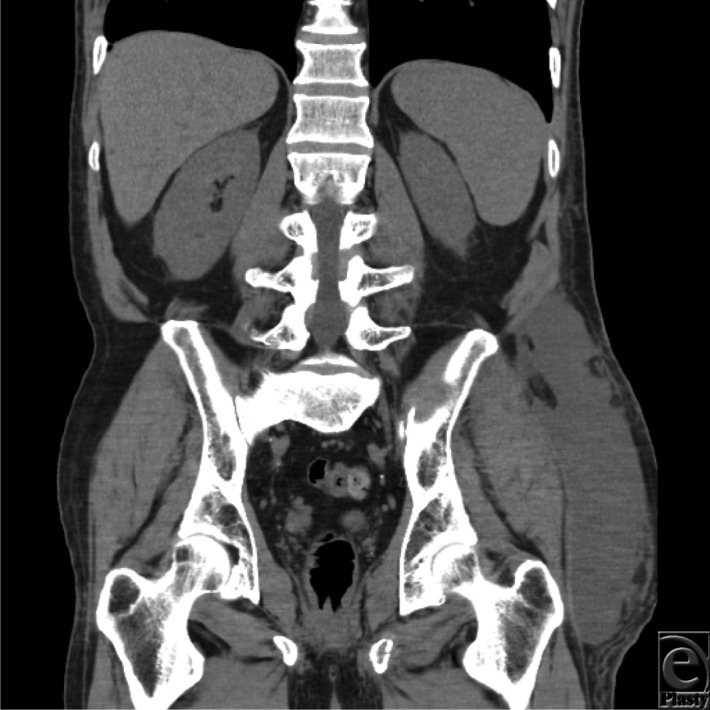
Magnetic resonance imaging coronal image of the Morel-Lavallée lesion in between the subcutaneous fat and the underlying fascia.
